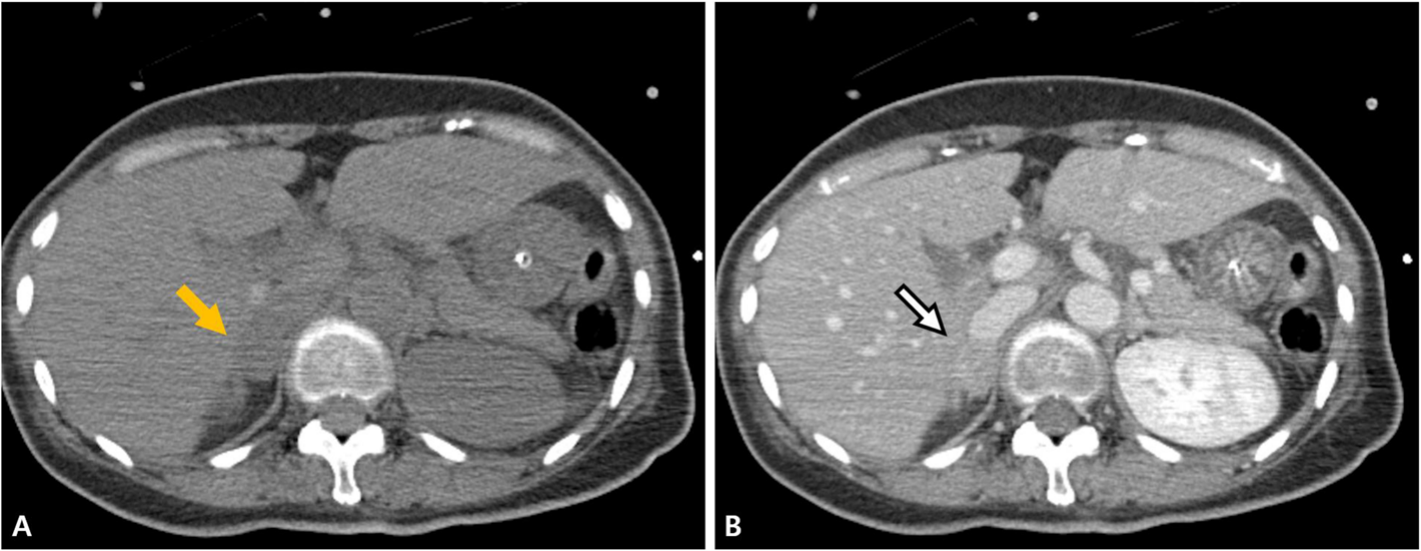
Abdominopelvic computed tomography (CT). A Pre-contrast abdominopelvic CT scan demonstrated a 1.7-cm-sized nodule (arrow), (B) contrast-enhanced CT showed enhancement (arrow) suggestive of an adenoma and confirmed by excisional biopsy with adrenalectomy
